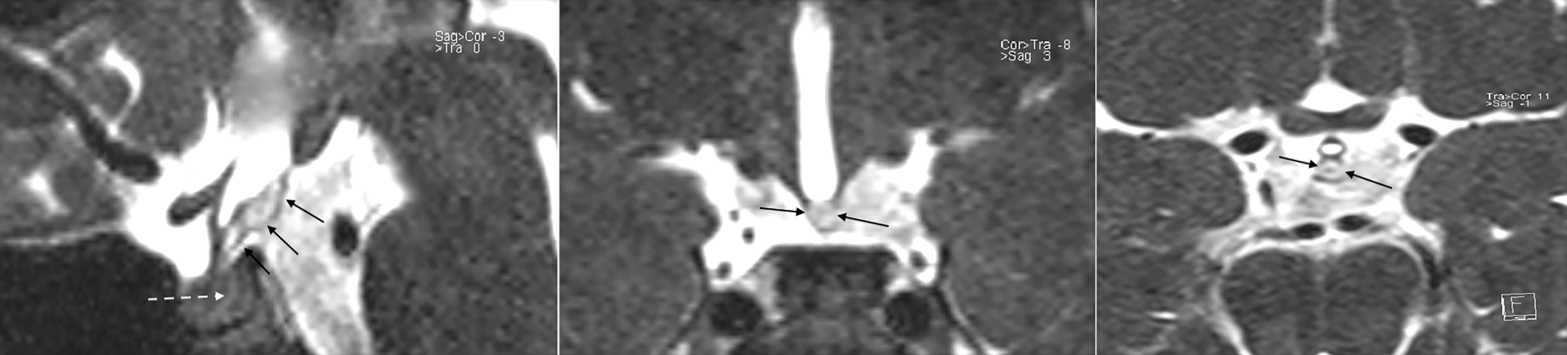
3D T2w SPACE sequences (sagittal, coronal and axial from left to right) show a high signal posterior to the tuber cinereum and poor contrast of the other tissues (continuous arrows); meaning the presence of a tissue in the suprasellar cistern, posterior to the tuber cinereum, different from neurohypophysis which remain hypointense as expected (dotted arrow). Fat has high signal on this sequence, suggesting being a lipoma of the tuber cinereum. The careful consideration of these sequences at the first MRI scan, furtherly to routine sequences, could have driven promptly to the 3D Fat Suppression T1w VIBE sequences for confirmation.
